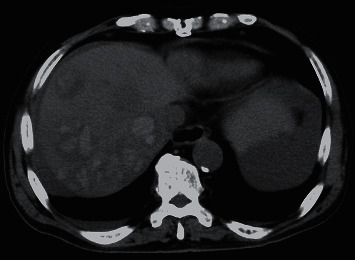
Nonenhanced computed tomography image. High-attenuation areas were observed in multiple HCCs considered to indicate multiple intratumoral hemorrhages.
